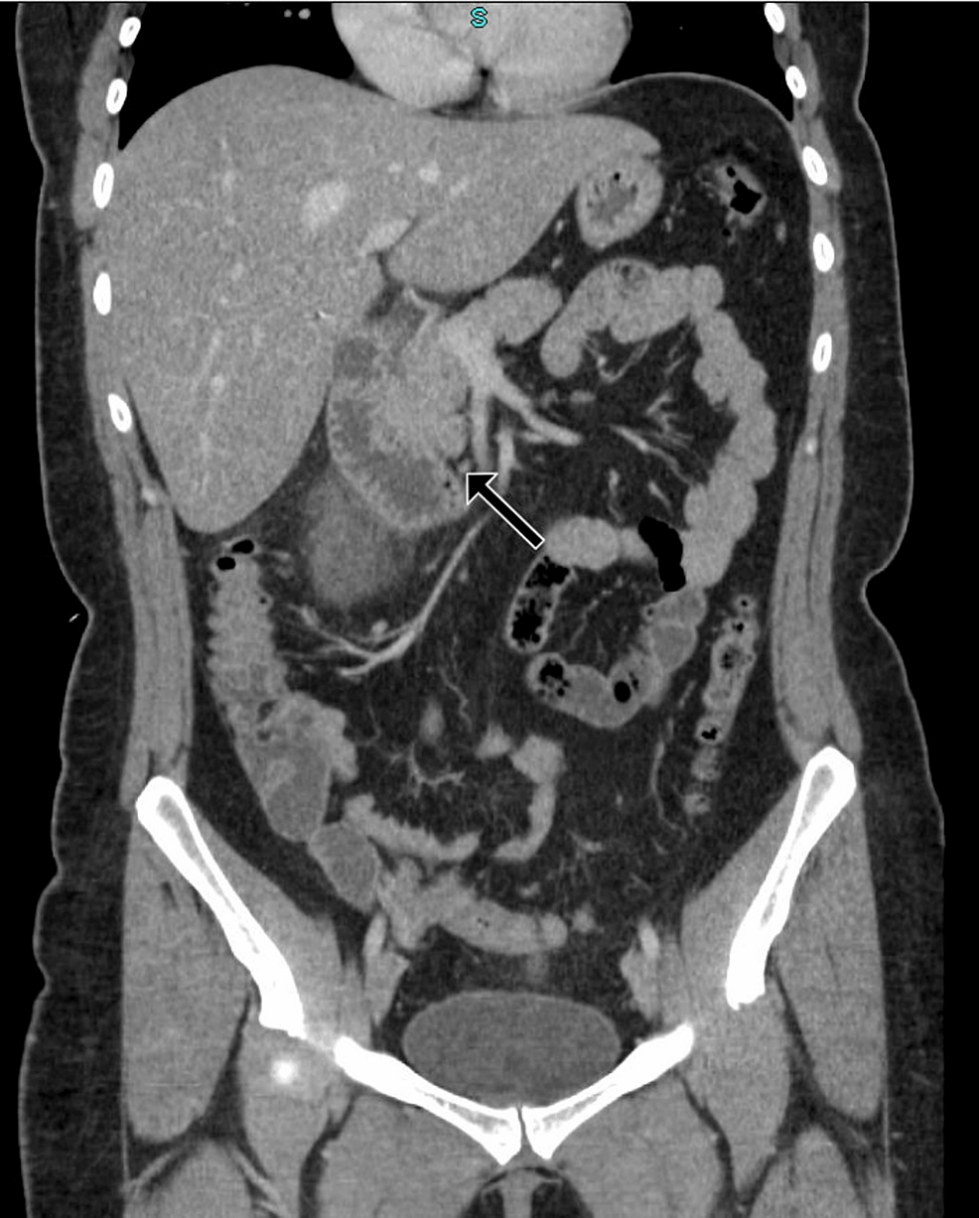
Coronal CT abdomen with groove pancreatitis Arrow pointing towards fluid interdigitating between the descending portion of the duodenum and pancreas. More fluid is identified inferior to the duodenum.
